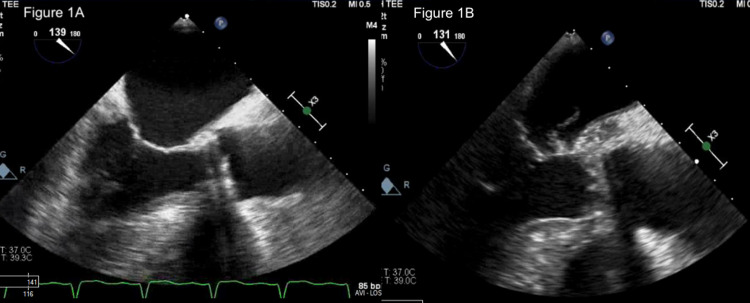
Transesophageal echocardiogram at the mid-esophageal view at baseline (A) and at five days (B).
